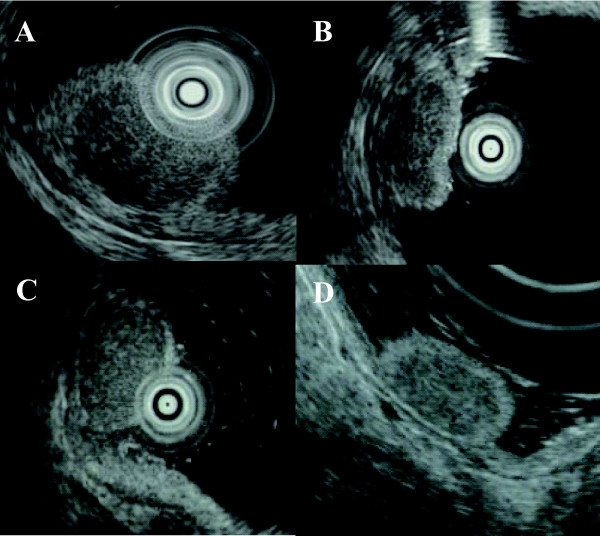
Endosonographic findings of the duodenal neuroendocrine tumors. A, B, C, D. Well-defined hypoechoic tumor confined to submucosal hyperechoic layer and the intact underlying hyperechoic muscularis propria (A: patient 1; B: patient 2; C: patient 3; D: patient 4).
